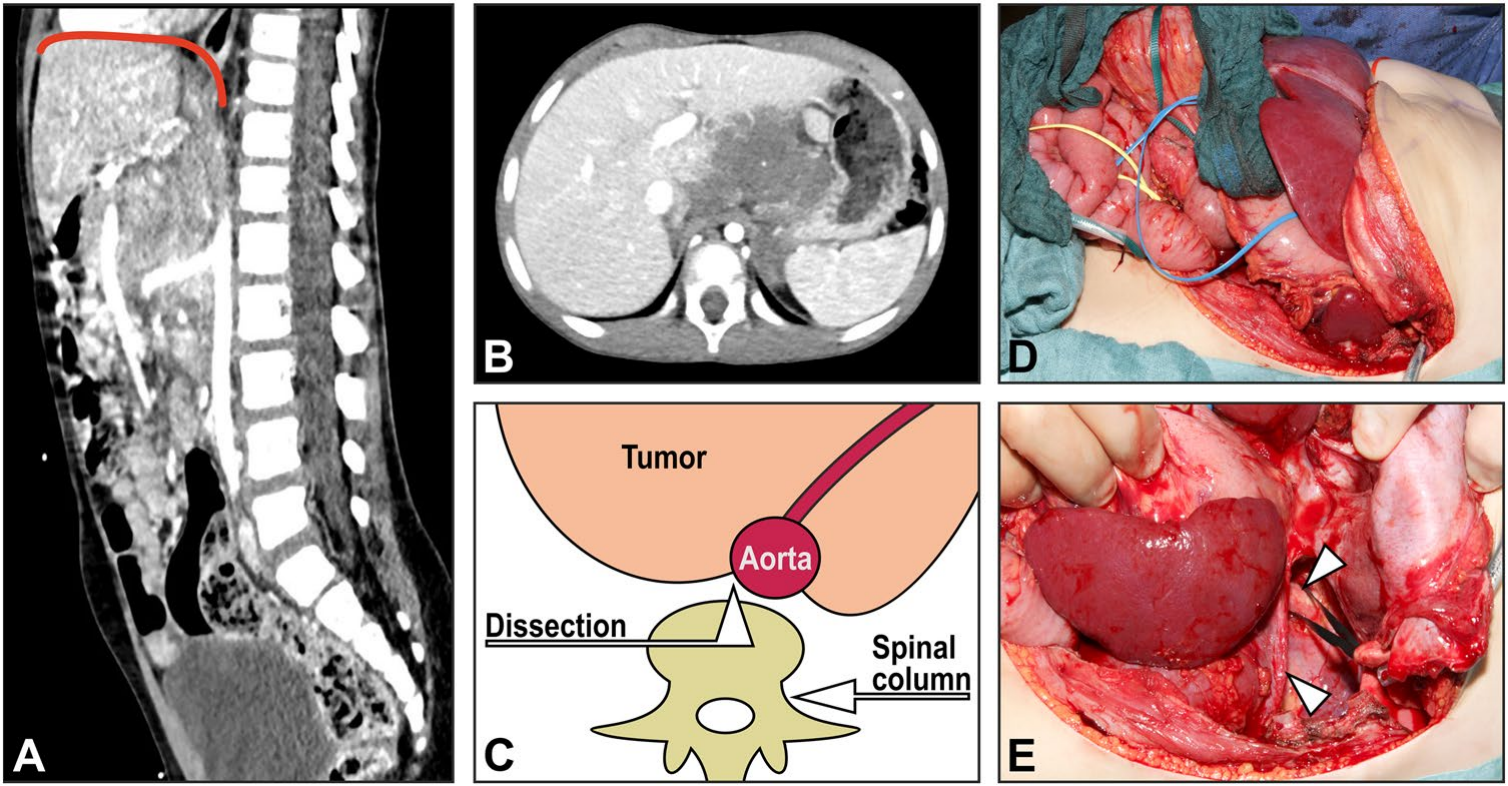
MRI scan images (A, B) of a patient with abdominal neuroblastoma extending up to the diaphragm (A, red curved line); The sketch (C) shows the principle of dissection technique, which always begin laterally on a tumor-free part of the aorta; using a thoraco-abdominal approach (D), the diaphragm (E, lower arrow) was opened and the thoracic aorta (E, upper arrow) was secured
